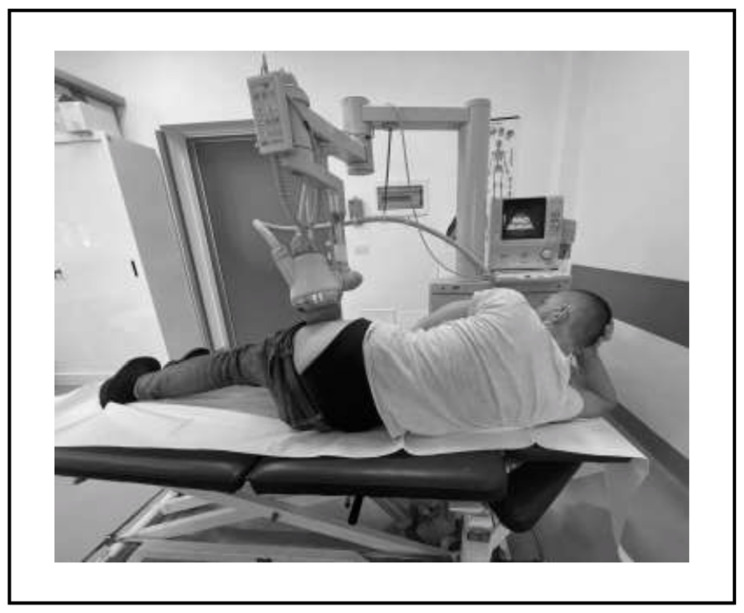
The patient is positioned on the side and the treatment probe is placed on the area to be treated, using an online ultrasound guide.
